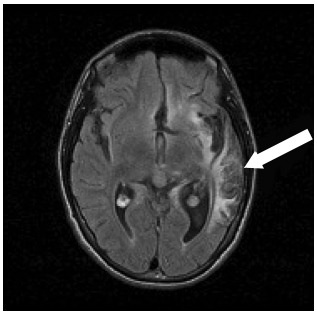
Magnetic resonance imaging performed in May 2007 showing areas of encephalomalacia with principal involvement of the left temporal lobe, and lesser involvement of the left frontal lobe. These represent areas typically affected by herpes simplex virus.
